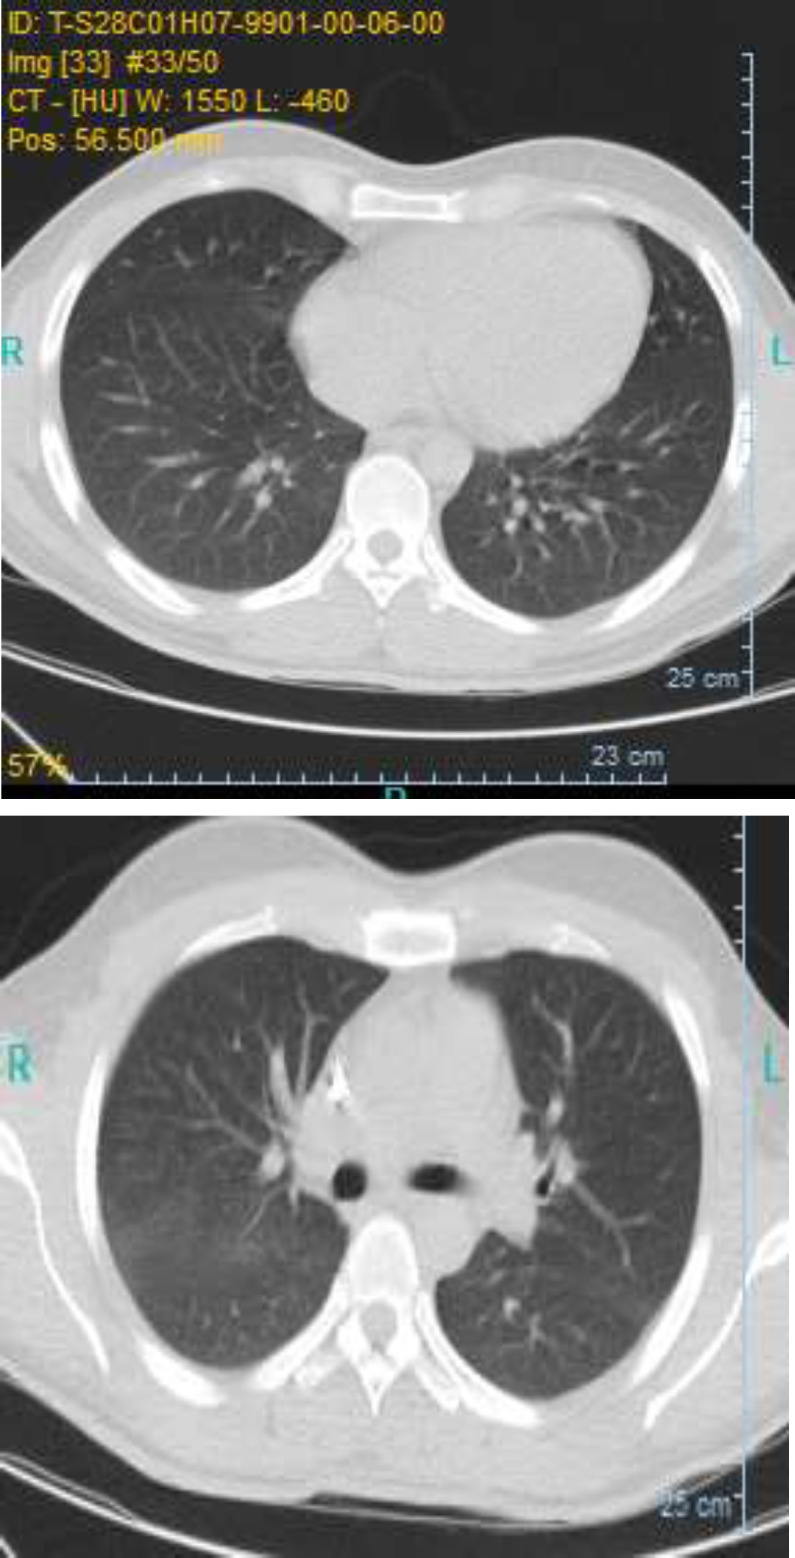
Chest CT-scans of case-patient with COVID-19 on April 2,2020after recovery
